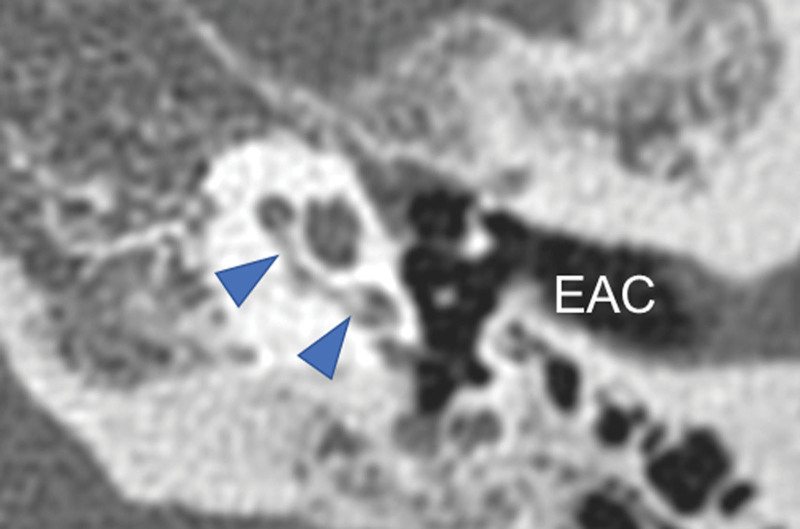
Temporal bone computed tomography, preoperative computed tomography revealed the ossification in the basal turn of the cochlea of the left ear (blue arrows). EAC: external auditory canal.
